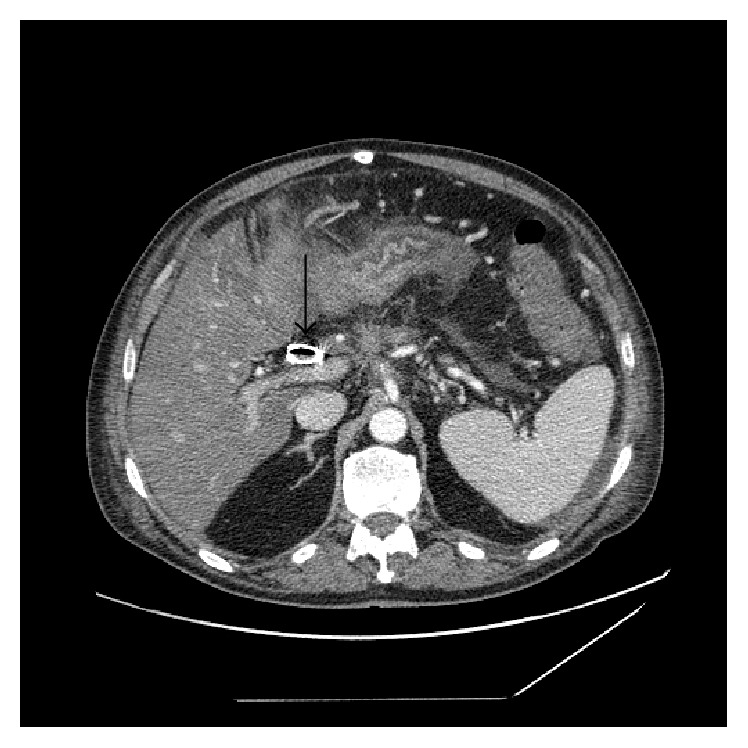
Abdominal contrast-enhanced CT, axial plane. Stented common bile duct is intimately close to the portal vein causing its minor compression.
